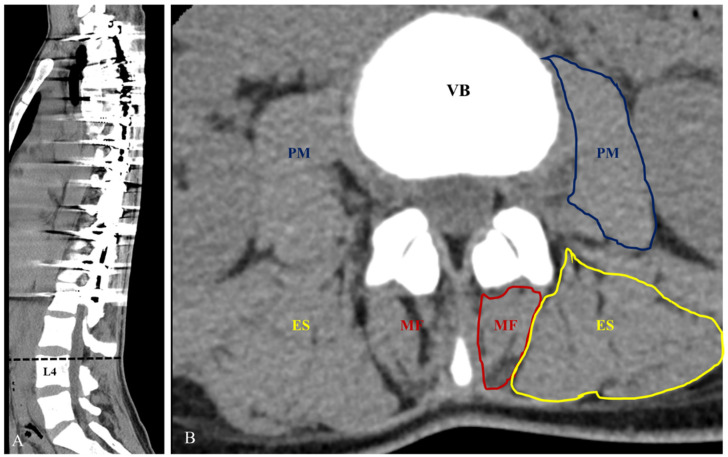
The cross-sectional areas (CSAs) of individual paraspinal muscles (multifidus (MF), erectus spine (ES), and psoas major (PM) muscles), and L4 vertebrae body (VB) were measured by assessment of axial computed tomography (CT) images. Measurements of the CSA of the paraspinal muscles were obtained at the level of the upper endplate of L4 by manual tracing (A,B).
